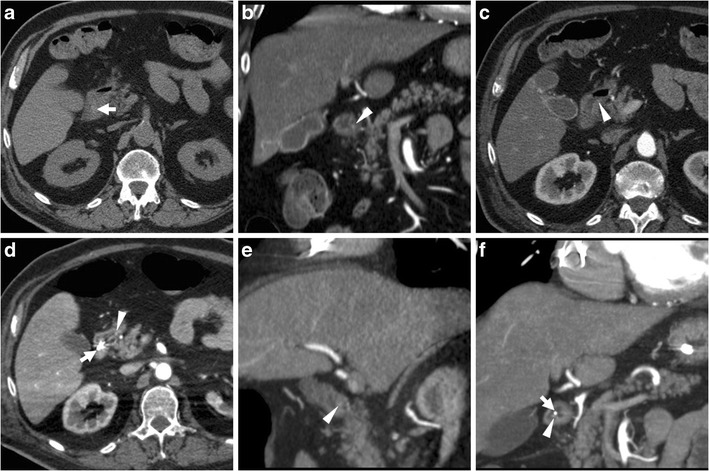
A 59-year-old female underwent emergency CT (a…c) because of hypotension and blood loss. Unenhanced scans (a) revealed subtle hyper attenuating content of proximal duodenum (thick arrows). Arterial-phase images (b, c) showed a dot-like mucosal enhancement (arrowheads) at dorsal aspect of duodenal bulb, which corresponded to bleeding ulcer and required endoscopic clipping and transfusions. Four days later, recurrent bleeding with melaena occurred: repeated CT-angiography (d…f) showed metallic clip (thick arrows) and thin jet-like intraluminal contrast extravasation (arrowheads), which was immediately treated with local epinephrine injection
